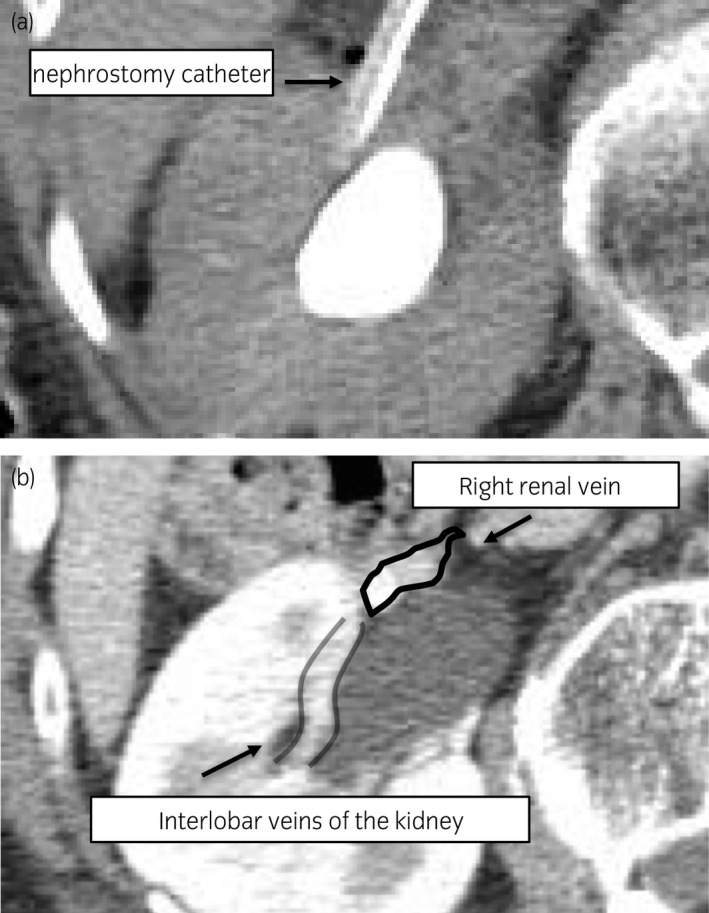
Abdominal CT scan. (a) Abdominal CT scan shows the nephrostomy catheter straying into the renal vein outside the renal pelvis. The contrast agent used in the angiography is seen only in the renal pelvis, and no extravasation of urine or bleeding from the renal pelvis is observed. (b) Abdominal CT scan before this event shows the interlobar veins of the kidney at the right ventral renal pelvis.
